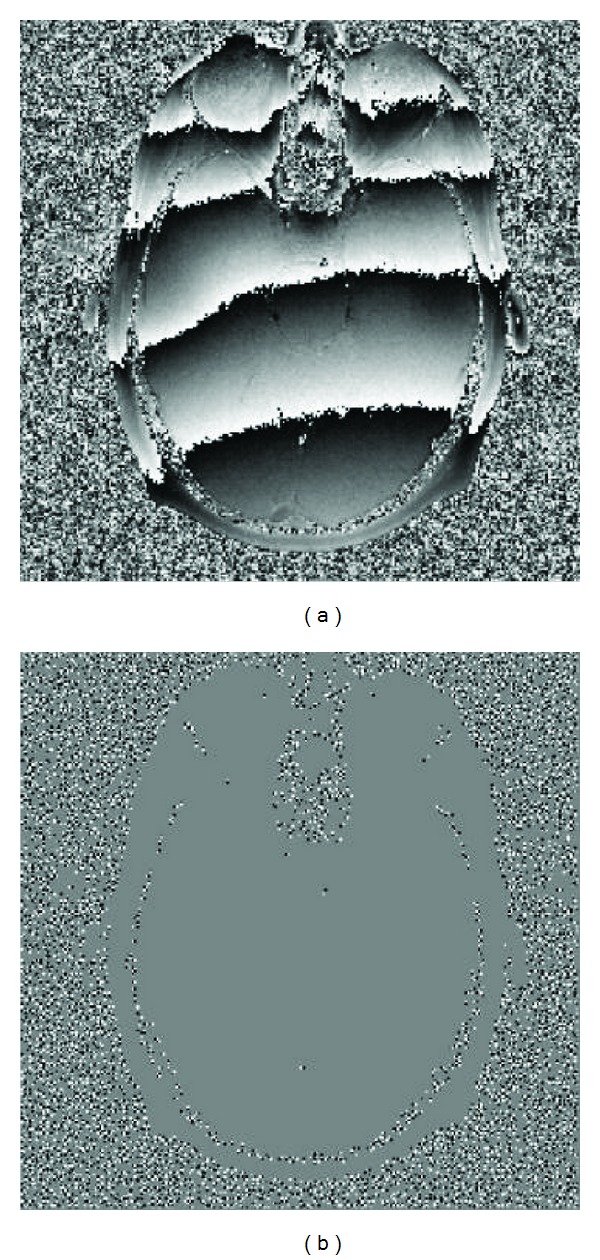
(a) A 256 × 256 MRI head phase image, (b) its corresponding residue distribution containing 9795 residues, 4904 positive polarity residues, and 4891 negative polarity residues.
